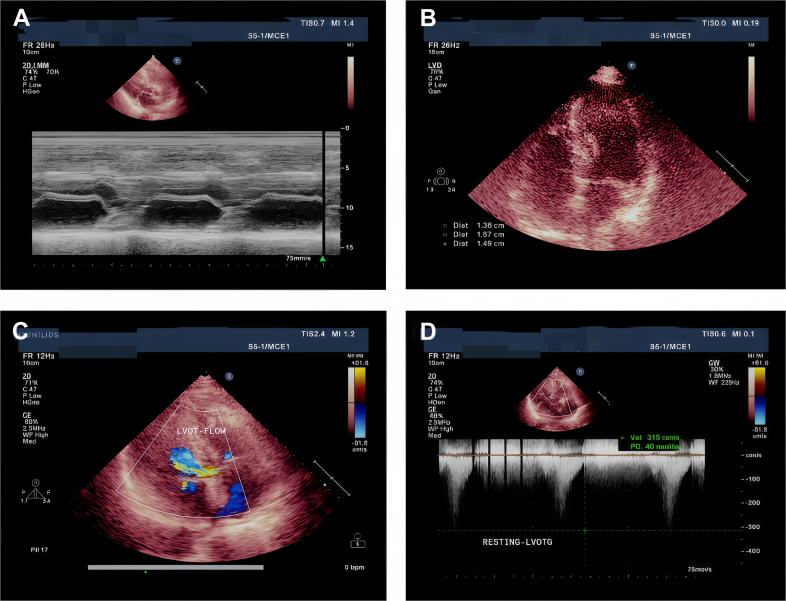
Comprehensive Echocardiogram Confirming Hypertrophic Obstructive Cardiomyopathy Integrated findings include systolic anterior motion of the mitral valve (A), asymmetric septal hypertrophy up to 18.7 mm (B), accelerated LVOT flow on color Doppler (C), and a continuous-wave Doppler-derived peak LVOT gradient of 40 mm Hg at rest (D). LVOT = left ventricular outflow tract.
